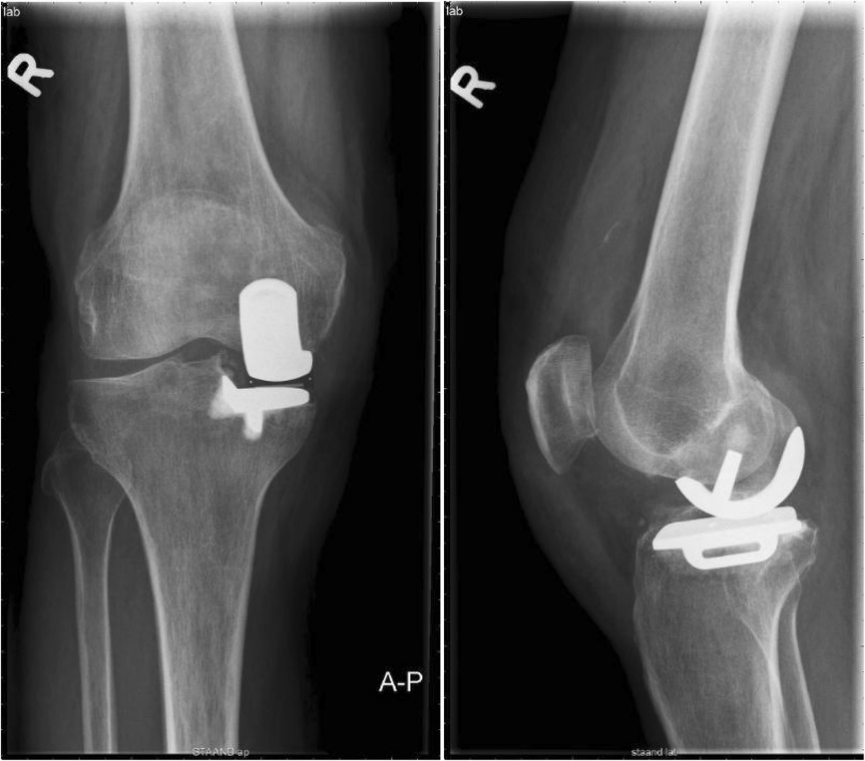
Postoperative anterior-posterior and lateral X-rays directly after the insertion of an Oxford unicompartmental knee prosthesis.
